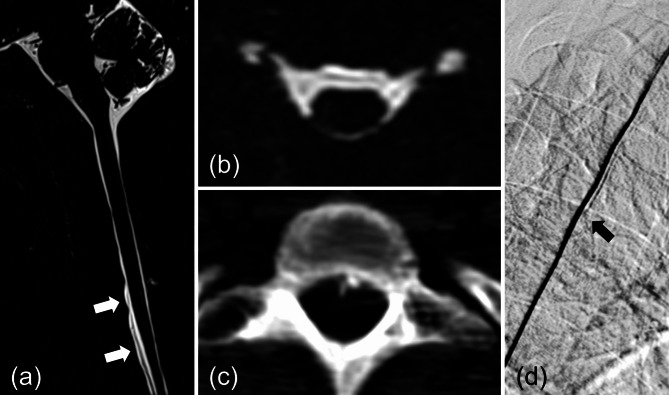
Imaging modalities used for patient evaluation and selection of EBP level. (a) Sagittal view of spine MRI with heavily T2-weighted fat-saturated imaging showing spinal longitudinal extradural fluid (SLEC). (b) Axial view of spine MRI showing ventral SLEC along with bilateral nerve root diverticula. (c) Noncontrast spine CT identifying the presence of discogenic microspurs. (d) Digital subtraction myelography (DSM) demonstrating a localised CSF leak at a mid-thoracic level
